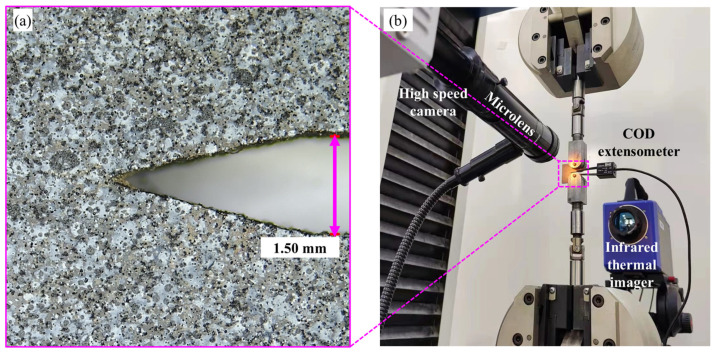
(a) A high-quality DIC surface featuring fine speckle patterns of varying sizes and gray levels from the tested CT sample. (b) Multi-field synchronous fracture testing system, including details on sample installation and instrument information.
